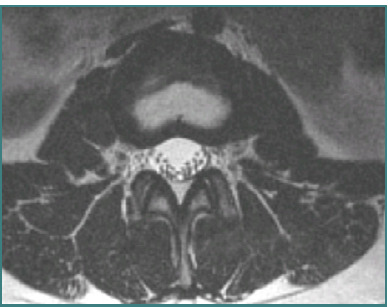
Axial view of L4-L5 level on MRI
